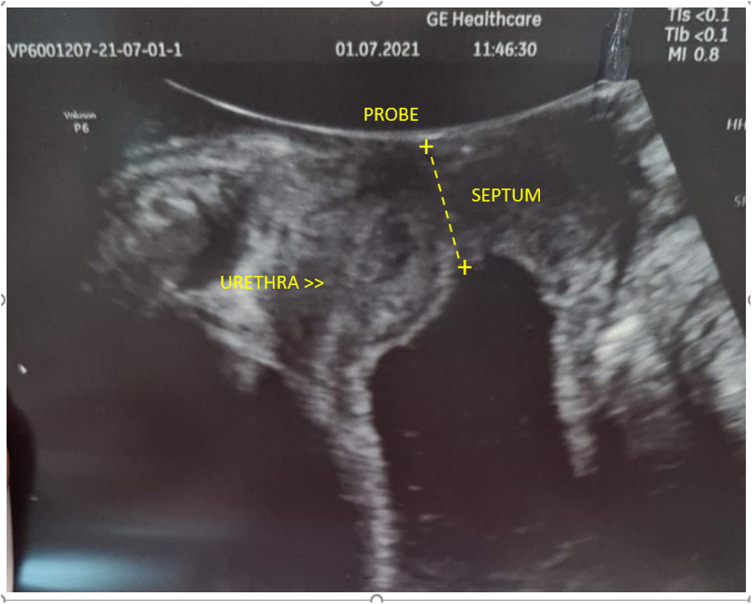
Transperineal ultrasound pictures: transverse vaginal septum approximately 1.2 cm in thickness appears as a hyperechoic structure. Below, it is seen as a large hypoechoic mass that responds to the accumulation of fluids in the vagina.
